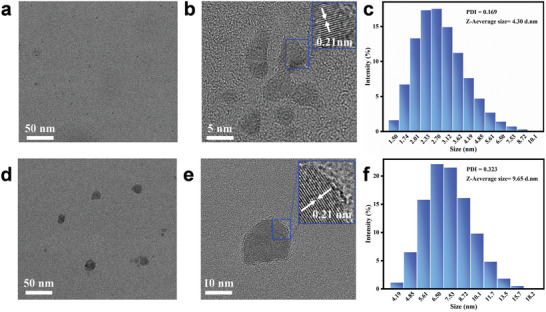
TEM images and particle size distribution of CPC‐CDs obtained after the ultrasonic treatment for 100 min (a,b; c) and 240 min (d,e; f).
